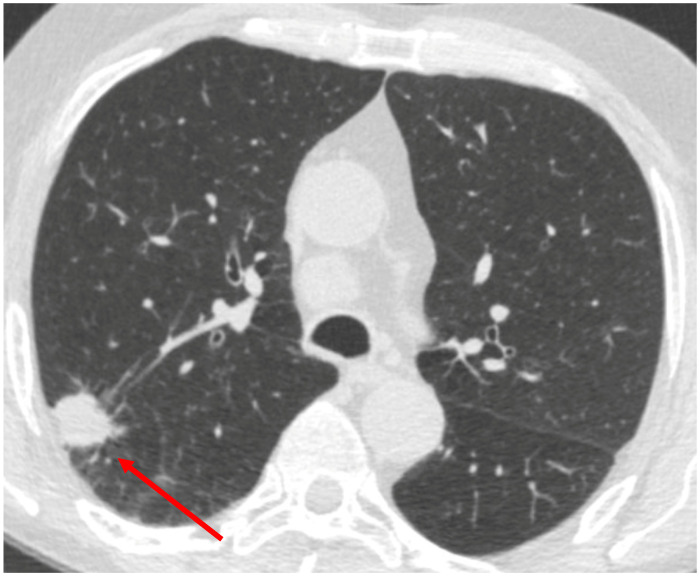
Representative CT image showing a spiculated right upper lobe nodule suggestive of malignancy, ultimately diagnosed with non-small cell lung carcinoma.
